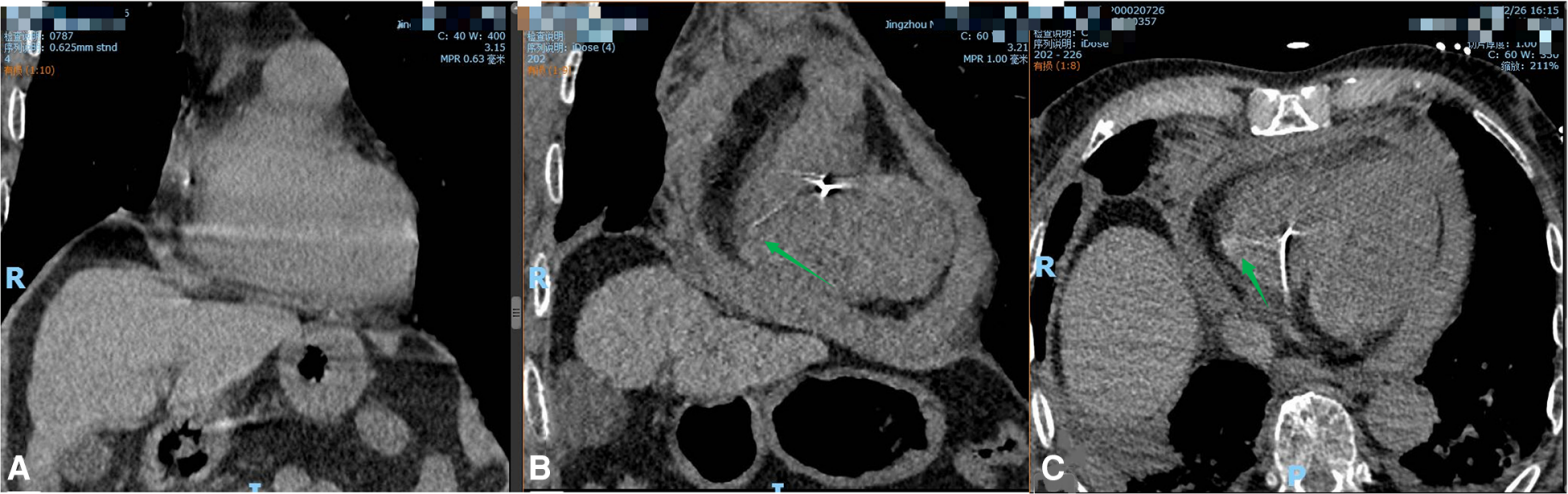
(A) Preoperative chest CT coronal plane; (B) postoperative chest CT coronal plane showed linear high-density shadow piercing the right ventricular wall, as shown by the arrow; (C) after operation, a linear high-density shadow punctured the right ventricle on the chest CT cross section, as shown by the arrow.
